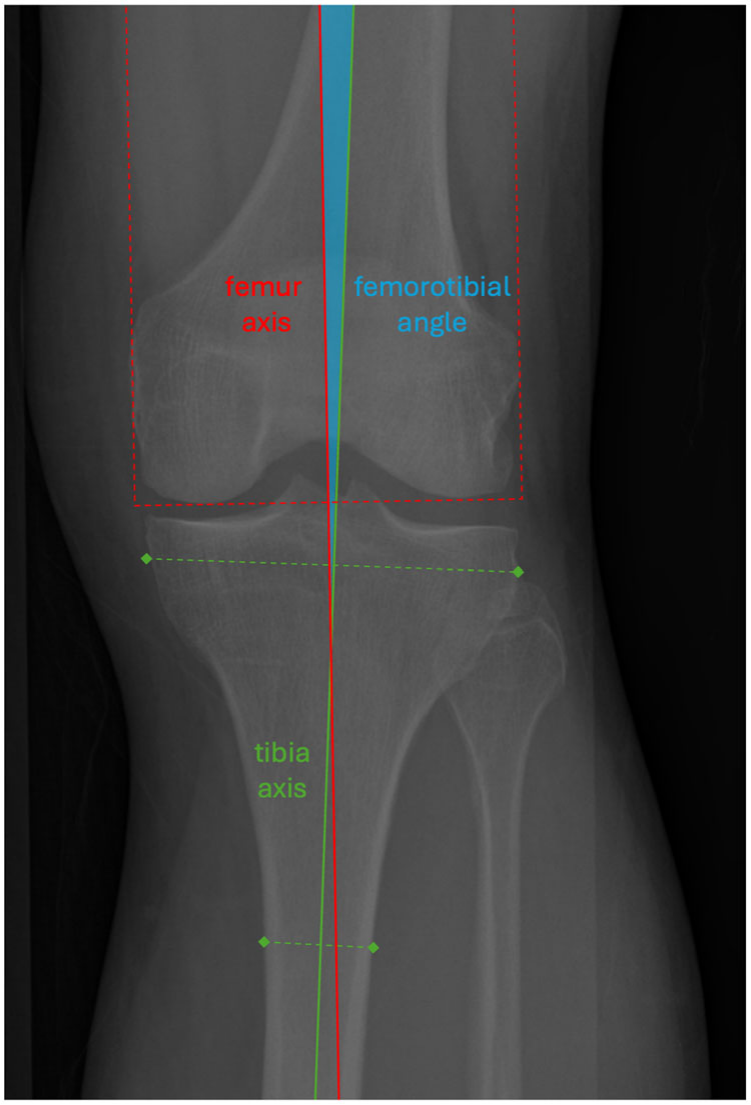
Radiograph showing the axes of the femur (red) and the tibia (green) for measurement of femorotibial angle (blue). The femur axis is perpendicular to a line tangent to the base of the femoral condyles and centred between the outer margins of the medial and lateral femoral epicondyles. The tibia axis is the line connecting the midpoints between the outer margins of the tibia at 1 and 10 cm below the lowest point on the tibial plateau. Varus alignments are negative.
